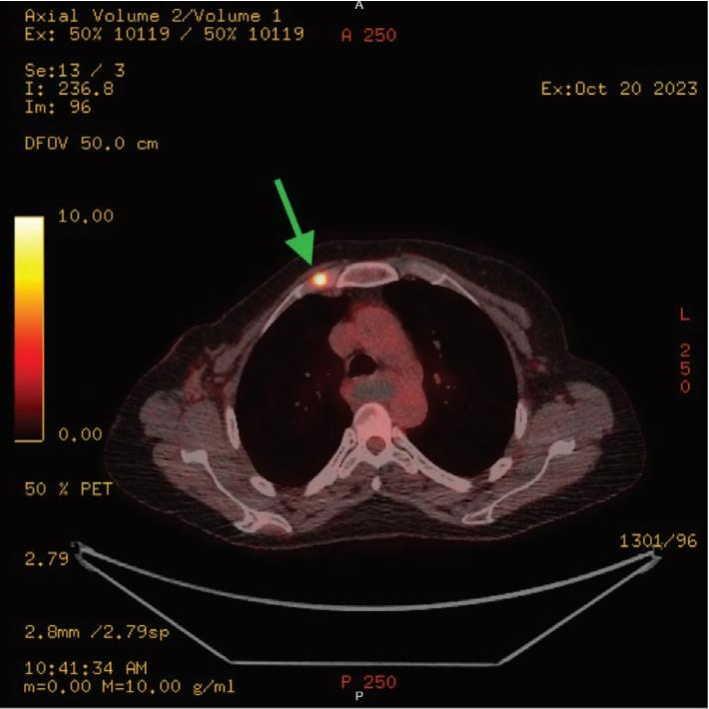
PET-CT, fused axial series, image 96 (20/10/2023). Image shows abnormal internal mammary chain node increasing in size (green arrow).
